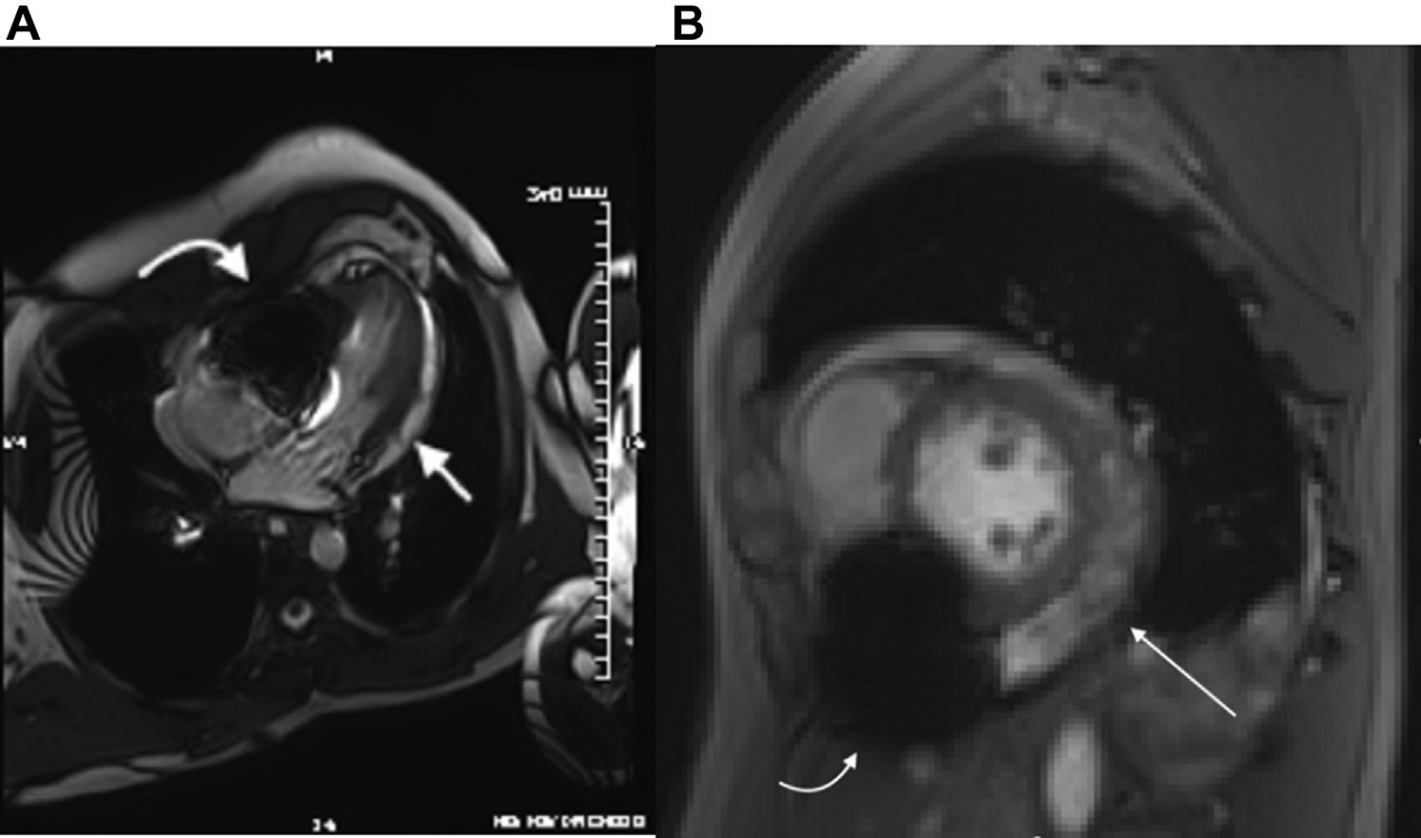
Cardiac Magnetic Resonance Steady-state free precession bright blood cardiac magnetic resonance images in (A) 4-chamber and (B) short-axis planes demonstrating the pericardial effusion (straight arrow) and a large area of susceptibility artifact with signal loss and distortion at the bottom of the right ventricle (curved arrow).
